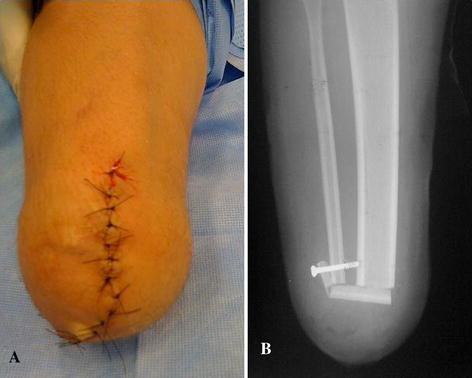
a X-ray showing a 3.5-mm screw fixing the distal pedicled strut graft osteoperiosteal flap to the distal fibula; b final stump
